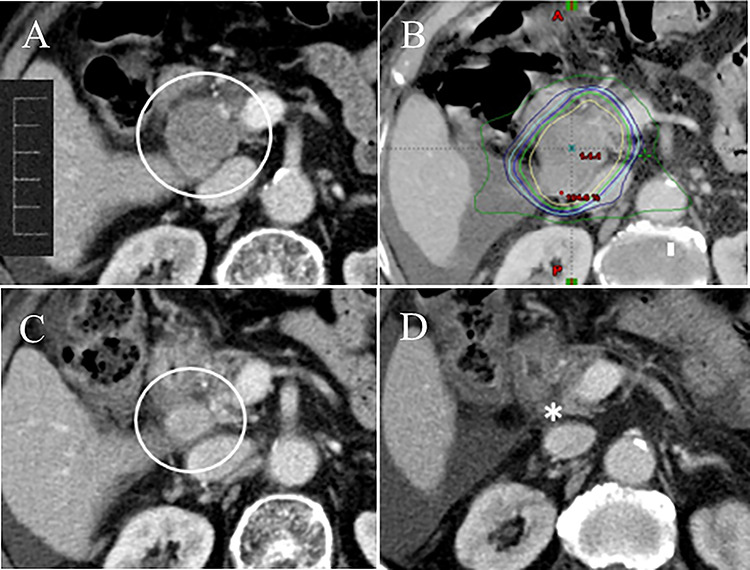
A 70-year-old female with HCC treated with TACE. (A) Abdominal CT scan showed an enlarged lymph node metastasis in the retropanceratic space with duodenal obstruction (circle). (B) Abdominal CT with dose distribution curves of SBRT delivered with IMRT. The innermost line was the 100% isodose line (yellow line). SBRT was administered with 45 Gy in 6 fractions (BED, 78.8 Gy_10_). (C) Abdominal CT at 2 months following the completion of SBRT showed a reduction of the metastatic node size (circle), and the RECIST response rate was partial response (PR). The duodenal obstruction was relieved. (D) The metastatic node disappeared on routine follow-up CT at 9 months following the completion of SBRT (asterisk).
